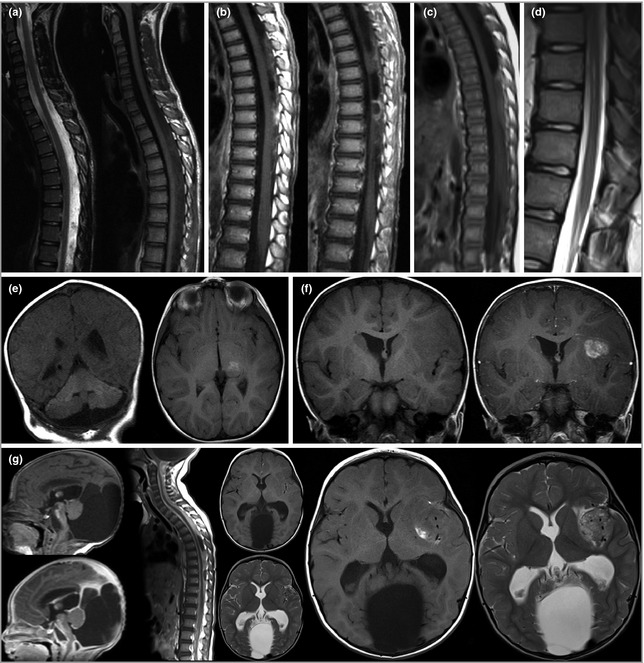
(a) Intradural extramedullary disease, presumed leptomeningeal, dorsal to the spinal cord. (b) Enhancing intradural extramedullary disease, presumed leptomeningeal, dorsal to the spinal cord. (c) Intradural extramedullary disease, presumed leptomeningeal, dorsal to the spinal cord. (d) Prominent central canal of the lower thoracic cord. These lesions have not been biopsied and therefore no exact diagnosis is available. However, in all cases shown the patients do not exhibit spinal symptoms or signs, and magnetic resonance imaging (MRI) appearances have been stable since birth, with follow‐up now at ages 5, 6, 10 and 16 years. (e) Extensive intraparenchymal melanosis of the cerebellum and focal melanosis of left thalamus. (f) Pre‐ and postgadolinium‐enhanced MRI showing meningioma in left sylvian fissure. (g) Pre‐ and postgadolinium‐enhanced MRI showing Dandy–Walker malformation with congenital leptomeningeal disease at 13 days. Further progression of leptomeningeal disease at 22 months. Intraparenchymal melanoma of left sylvian fissure at the age of 24 months, which appears to have developed from leptomeningeal infiltration, although this is not always the case.
